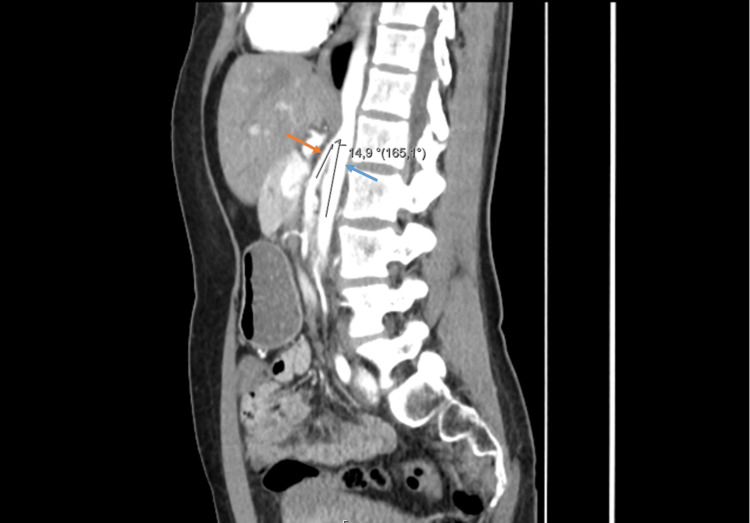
Sagittal CT image at the level of the origin of the SMA (orange arrow) from the aorta (blue arrow) showing a aortomesenteric angle of 14.9° CT: Computed Tomography; SMA: Superior Mesenteric Artery
